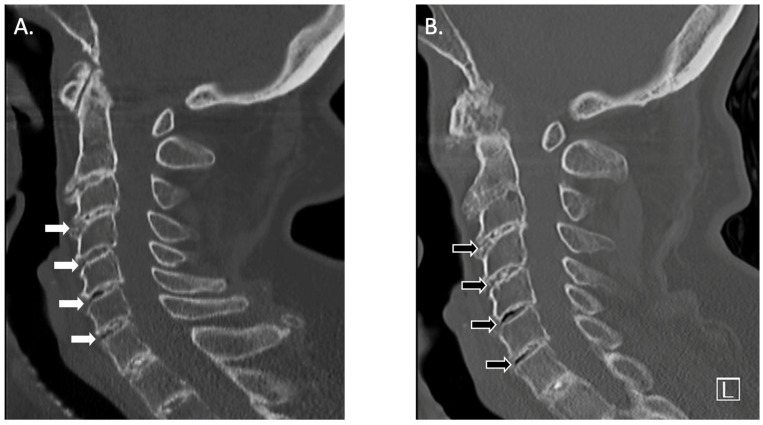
(A) Sagittal computed tomogram of the cervical spine of the patient taken 6 years ago when she was 77 years old. Destruction of intervertebral disc cartilage is apparent (white arrows). (B) Sagittal computed tomogram of the cervical spine of the same patient five years later, when she was 82 years old, showing progressive disc degeneration (black arrows) resulting from advancing disc cartilage destruction and reactive bone formation over the 5-year period. Vacuum phenomena are also apparent in the inferior two disc spaces.
